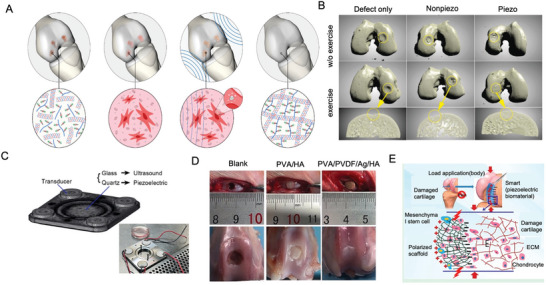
Piezoelectric materials promote cartilage repair. A) Ultrasound (US) stimulation, utilizing nanomaterials piezoelectric to stimulate cartilage tissue regeneration. Reproduced with Permission.^[^
